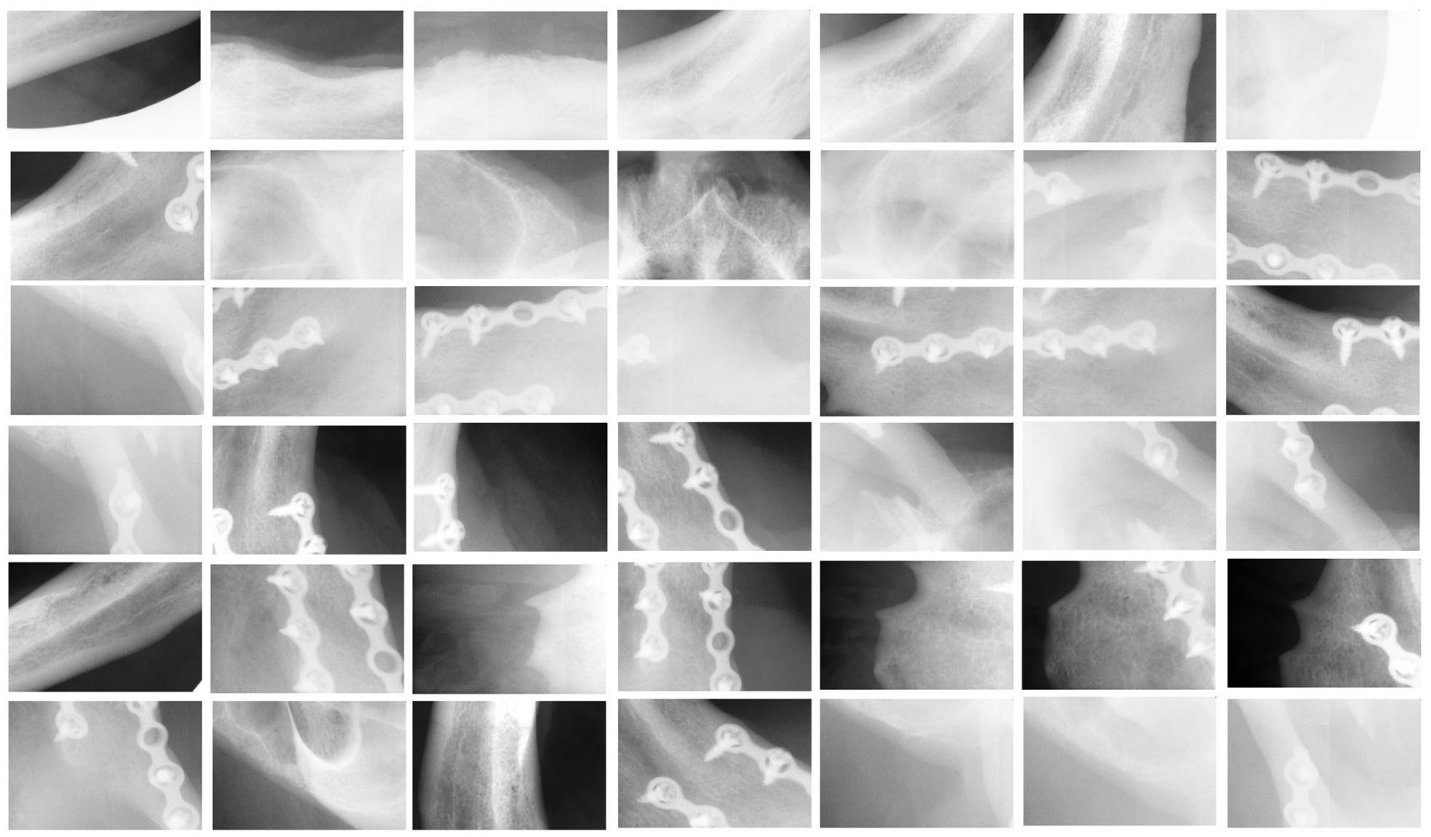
Forty-two periapical X-ray images obtained using a portable X-ray device coupled with a digital radiological sensor.
